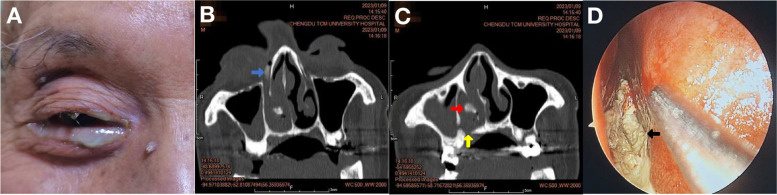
The patient's preoperative eye appearance, CT images of the sinuses, and nasal endoscopic images. A The patient showed obvious tearing and purulent discharge in the right eye on admission. B Horizontal CT images showed high-density signals of the right dacryocyst and part of the nasolacrimal duct (blue arrowhead). C Horizontal CT images showed bone discontinuities in the right mandible (yellow arrowhead) and a soft-tissue-density shadow in the right nasal cavity with a high-density shadow (red arrowhead). D nasal endoscopy shows many yellow and white nasal stone-like foreign bodies filled the right common and inferior nasal passages (black arrowhead)
